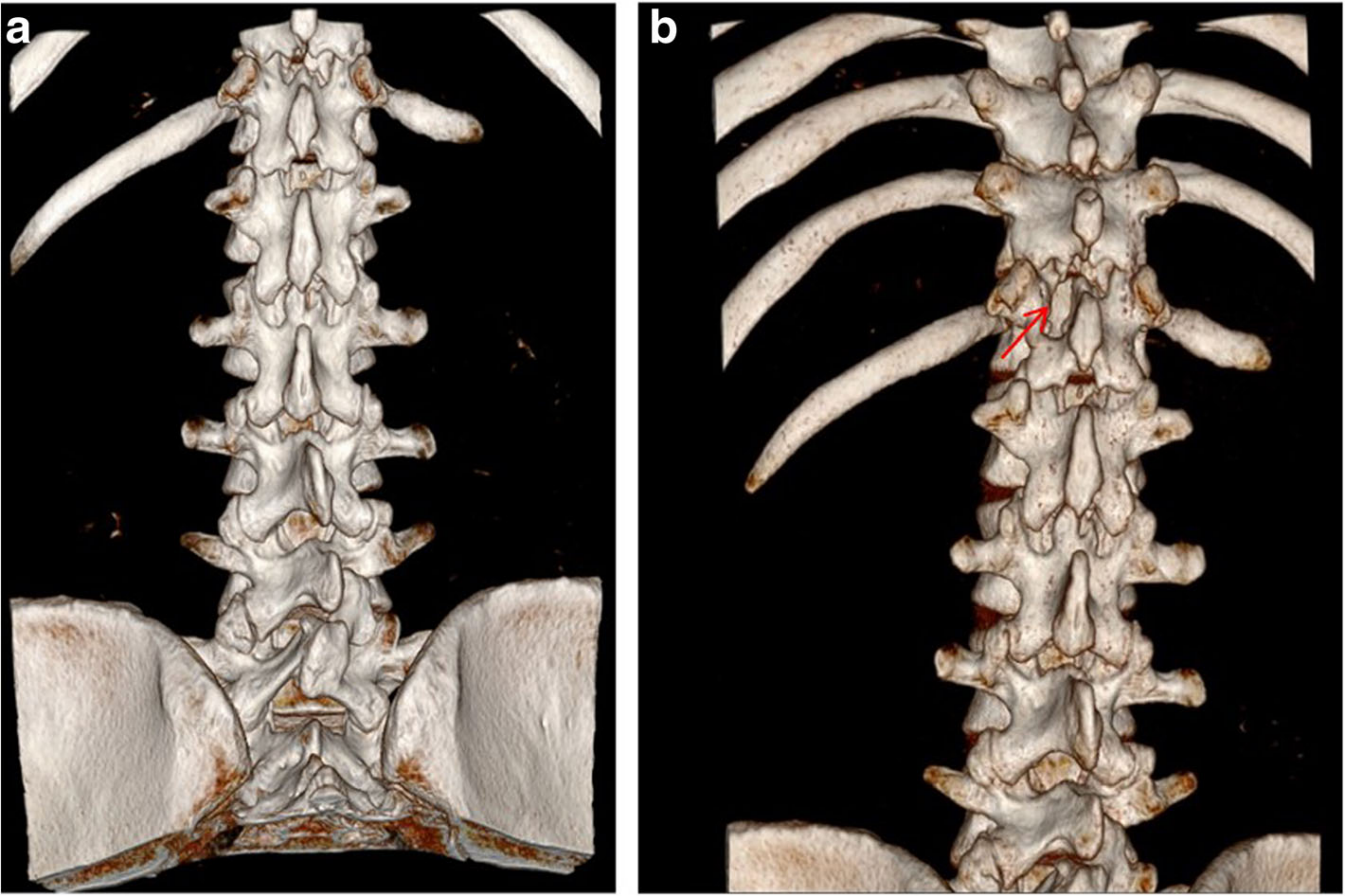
a The pre-operative 3-D reconstructive computed tomography (3-D RCT); b The post-operative follow-up 3-D RCT showing a small defect of right lamina (red arrow)
